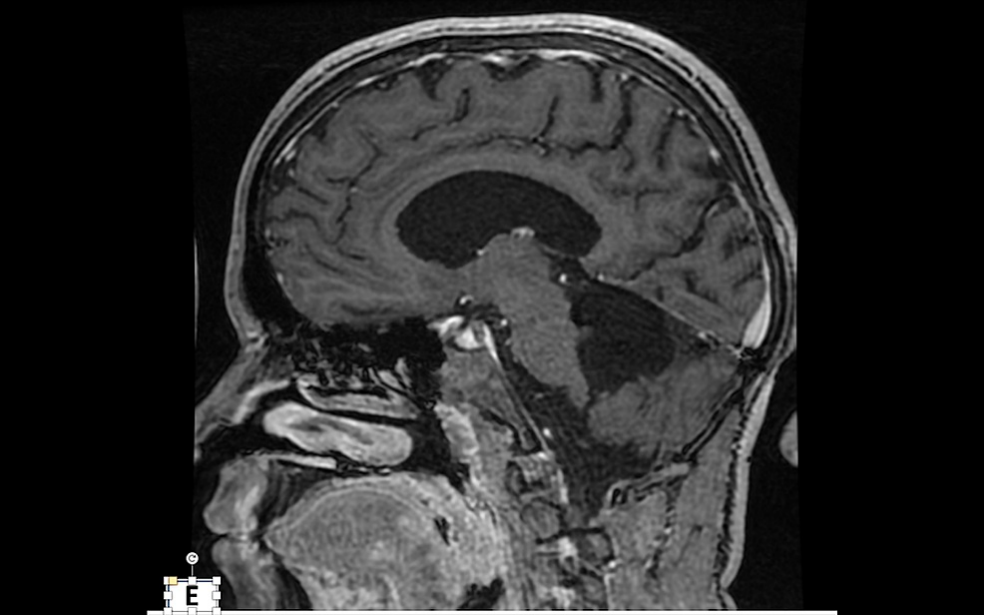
MRI scan of the brain Sagittal T1 with contrast taken 2 years after surgery showing no residual or recurrent tumor
